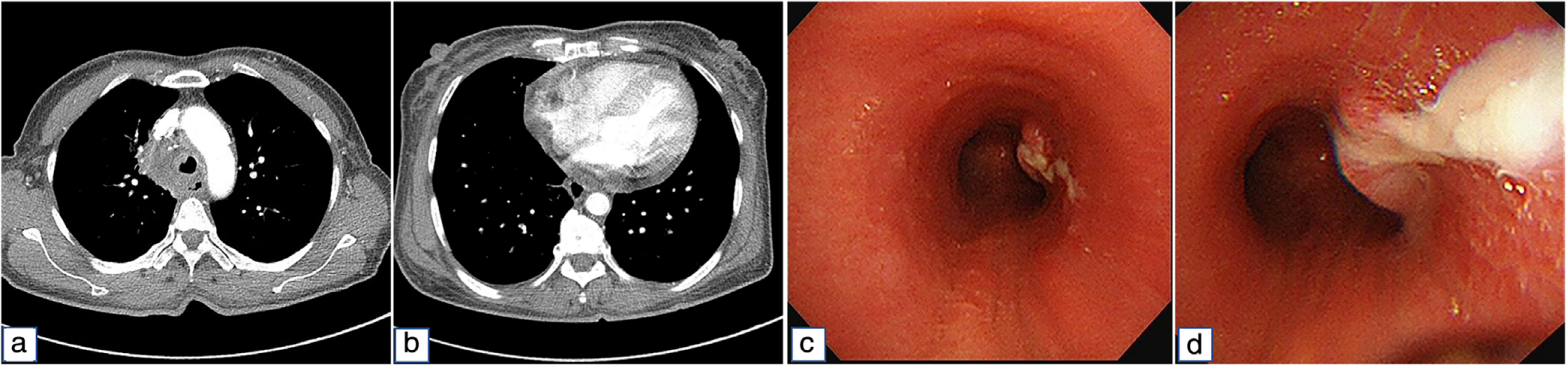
(a, b) Computed tomography (CT) revealed enlargement of mediastinal lymph nodes (a) and pericardial effusion (b), suggestive of mediastinitis. (c, d) Pus draining through the fistula opening in the right lateral aspect of the distal trachea during bronchoscopy
